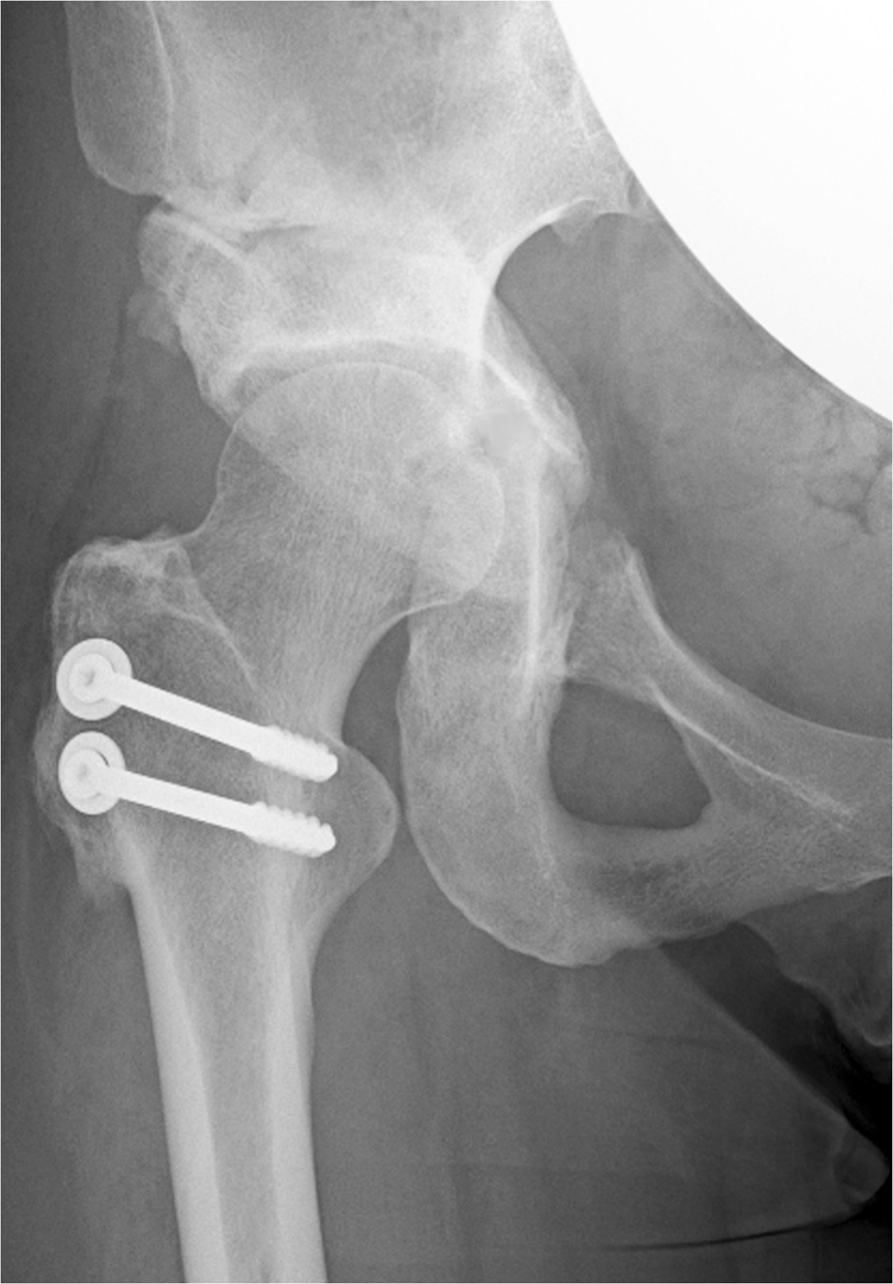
A radiograph of the same patient made 2 years after osteotomy showing solid union of the fracture. The right buttock pain continued for 3 months and then decreased. The patient reported no hip pain. The Harris hip score was 98 points at the time of follow-up.
